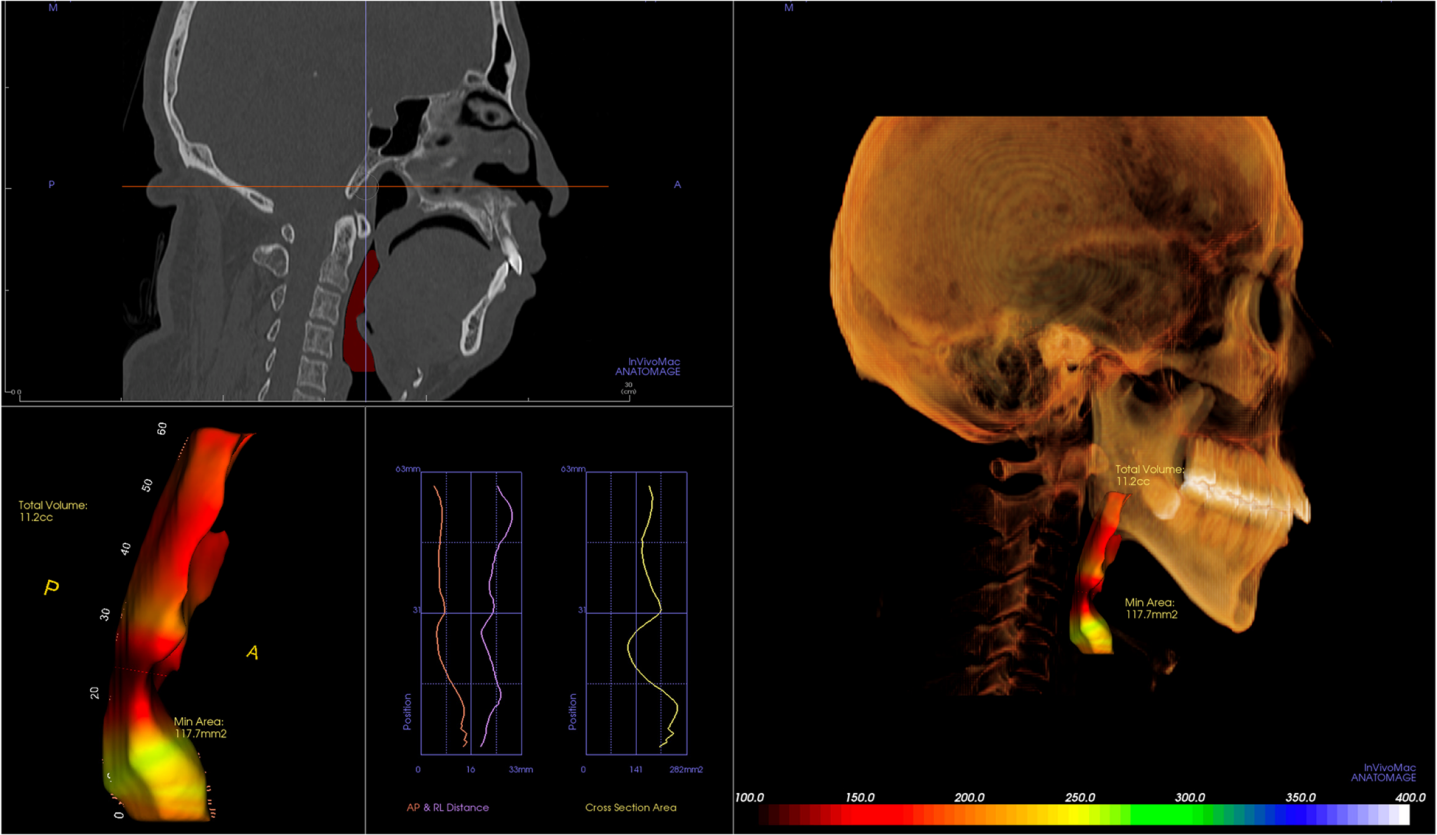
The volume and shape of the airway can be reconstructed from the CT dataset. Minimal cross-sectional area can be identified and this can be a candidate for a target of a surgery
